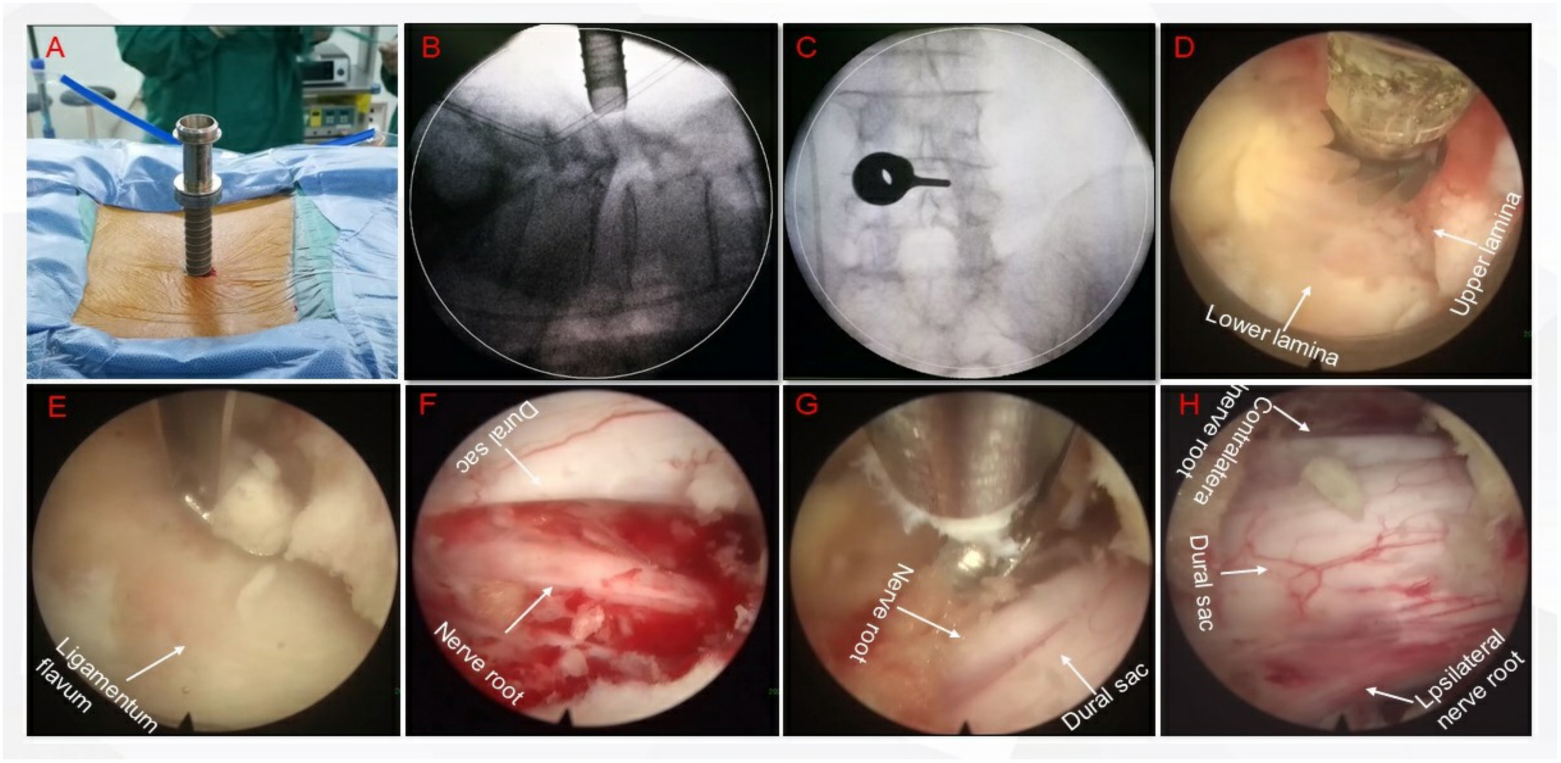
A representative case. (A) Place the working channel; (B) Lateral X-ray fluoroscopy confirms that the channel is facing the intervertebral space; (C) Positive X-ray fluoroscopy confirms that the channel is located inside the facet joint; (D) Clean up the soft tissue on the surface of the lamina, confirm the lamina space and upper and lower lamina; E. Remove the lamina and expose the ligamentum flavum; (F) Decompression of the nerve root on the same side, ranging from the initial segment of the nerve root, down to the inner edge of the pedicle, explore the Ipsilateral nerve root canal to achieve at least 270° decompression of the nerve root canal; (G) Decompression of the contralateral nerve root; (H) After the decompression, the dural sac and bilateral nerve roots are visible.
